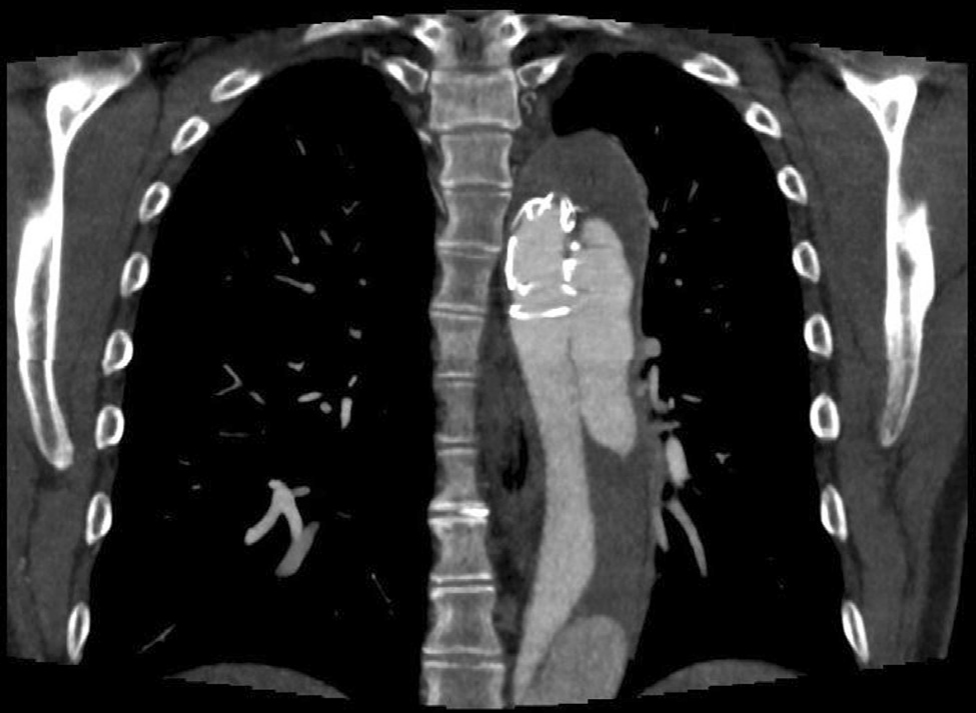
Representative computed tomographic scan of a distal stent-graft induced new entry (dSINE) that developed 1 year after frozen elephant trunk implantation.
